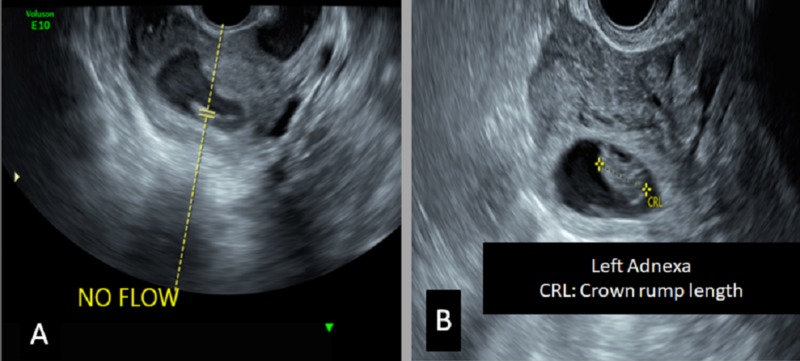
Transvaginal ultrasound A) A single nonviable intrauterine gestational sac is noted; B) There is evidence of the gestational sac hosting fetal pole in the left adnexa as well. These findings confirm the diagnosis of heterotopic pregnancy.
